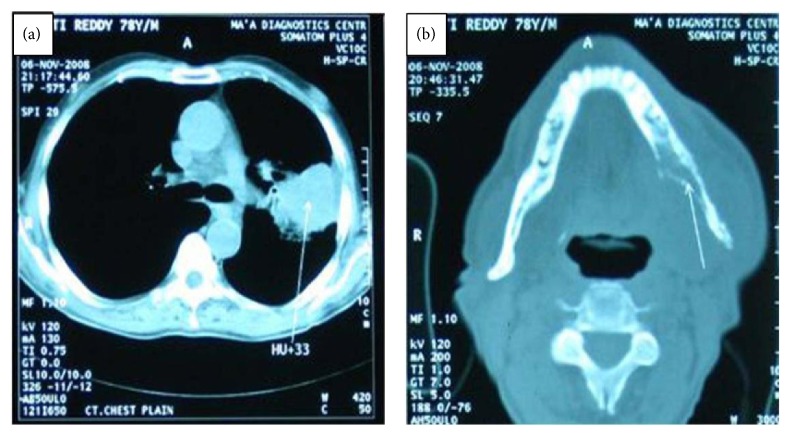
(a) CT chest revealed lesion in the left lung. (b) Axial sectional study of CT mandible revealed osteolysis of left body and ramus of the mandible with adjacent soft tissue mass.
